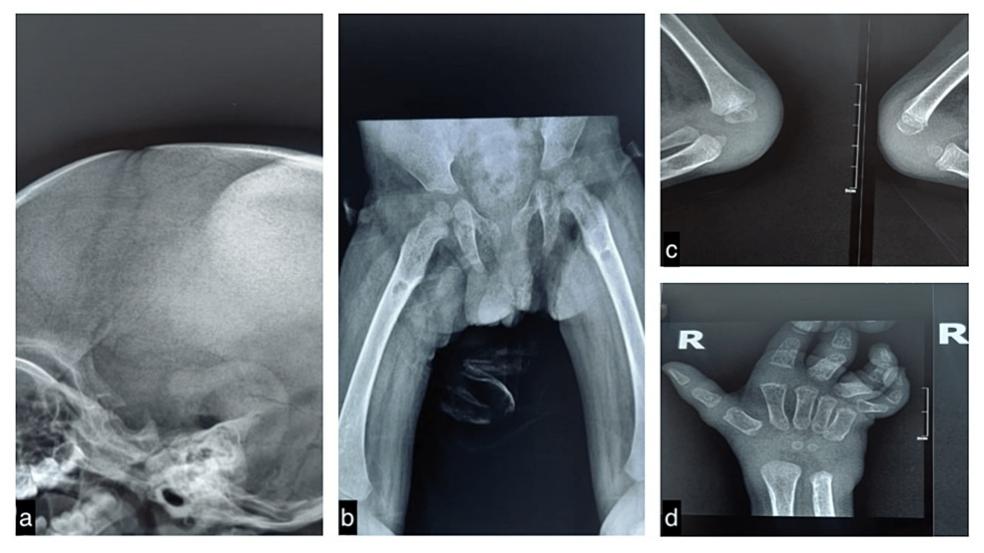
The skull radiograph shows a large soft tissue lesion in the parietal and occipital regions with no obvious signs of involvement of the underlying bone (a). Multiple symmetrical osteolytic areas in the proximal third of femoral shaft (b). Symmetrical osteolytic lesions with narrow zones of transition at the proximal tibial diaphysis and soft tissue opacities (c). Extension at the metacarpophalangeal joints, flexion at the interphalangeal joints, and soft tissue opacities around the wrist joints (d).
